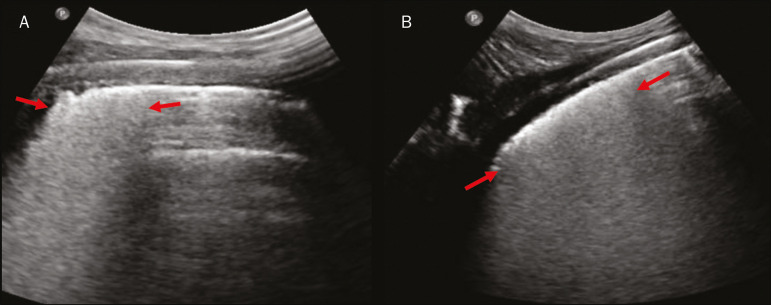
The chest ultrasound view demonstrates coalescent pleural B-lines in a patient with COVID-19. A,B: White lung appearance (arrows). On CT, this finding corresponds to ground-glass opacities.
